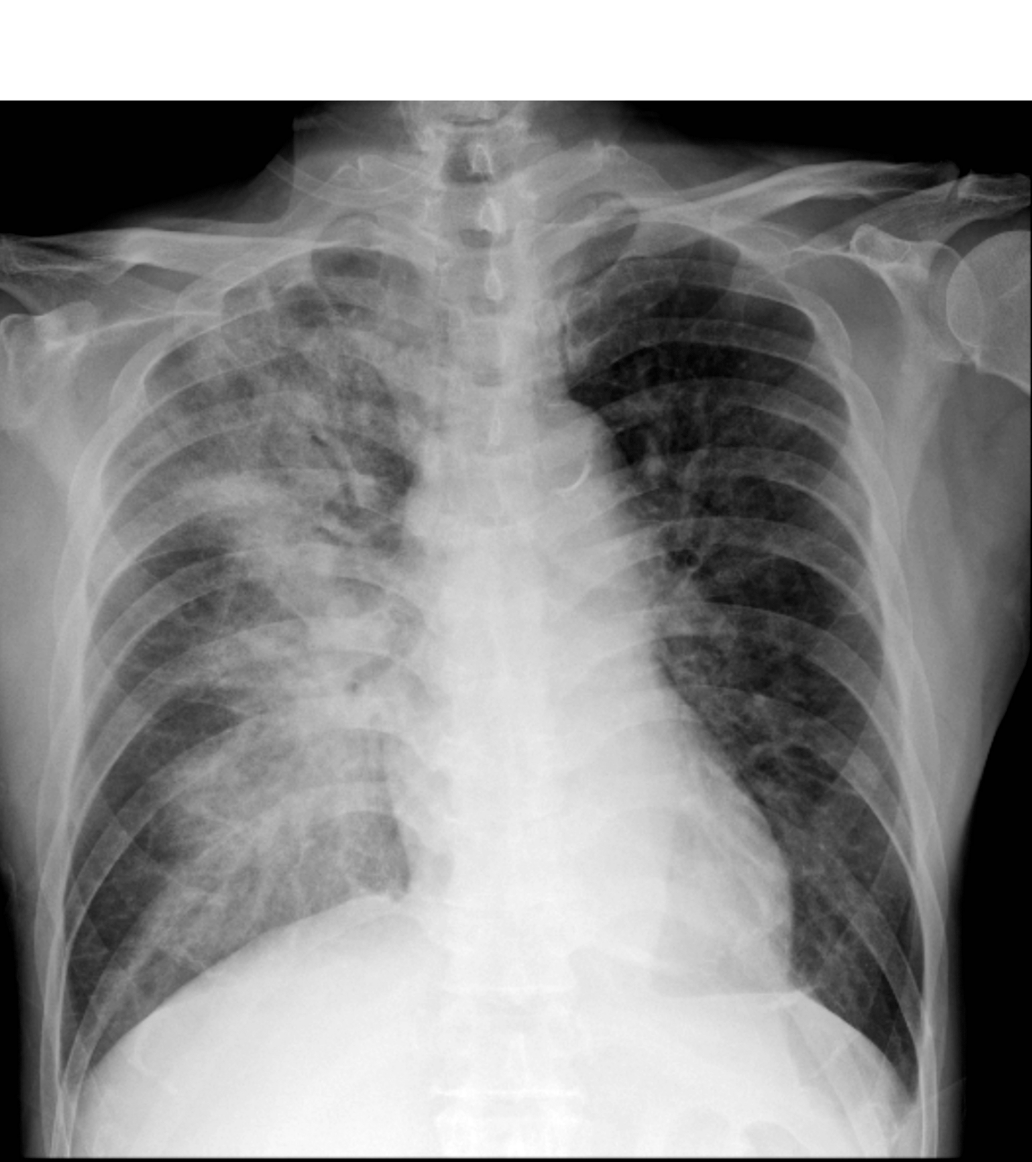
Chest X-ray The chest X-ray shows ground-glass opacities in the right lung field, suggesting pulmonary congestion secondary to acute mitral regurgitation.
